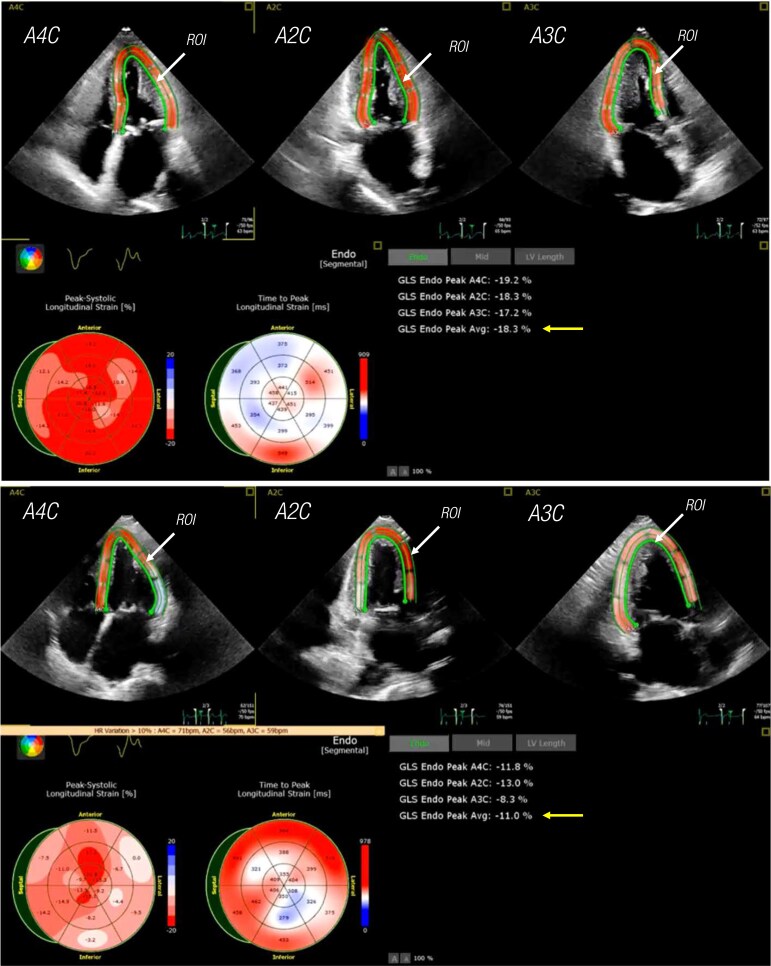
Global longitudinal strain (GLS) assessed by speckle-tracking echocardiography (STE). Global longitudinal strain was derived from apical 4-chamber (A4C), 2-chamber (A2C), and 3-chamber (A3C; apical long-axis) views. The myocardial region of interest (ROI) was defined by endocardial and epicardial contours. The bull's-eye (polar) plot summarizes peak systolic longitudinal strain, with central, middle, and outer rings corresponding to apical, mid-ventricular, and basal segments, across inferior, anterior, lateral, and septal regions. More negative strain values indicate better myocardial contractility. Color coding reflects strain magnitude (red/dark = more negative, better strain; yellow/green = intermediate; and blue/pale = less negative, worse strain). Diffuse color attenuation suggests global left ventricular dysfunction, whereas focal blue regions indicate regional dysfunction. The numeric GLS value represents the average strain across all segments. Abnormal GLS was defined as >−16% for the left ventricle (LV) and > −17% for the right ventricle. Representative examples show a patient with preserved LV GLS (−18.3%; upper panel) and a patient with markedly reduced GLS (−11%) consistent with severe LV systolic dysfunction (lower panel).
